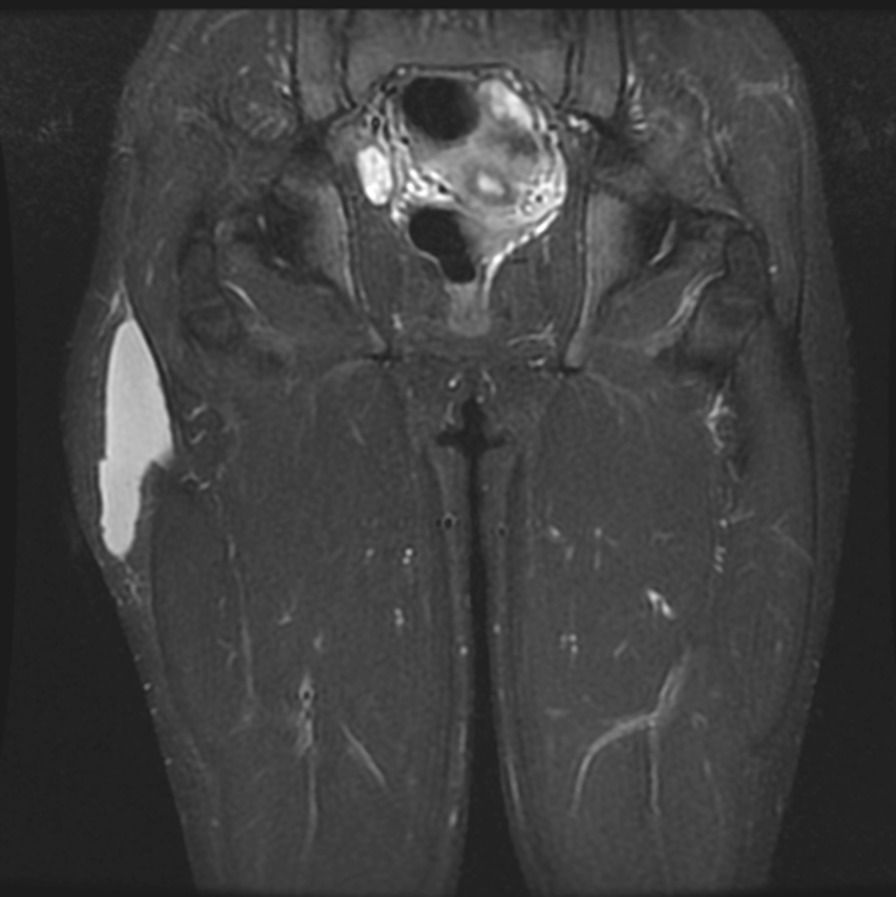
Coronal MRI STIR image that shows a chronic hematoma adjacent to the iliotibial tract, Morel-Lavallee lesion
